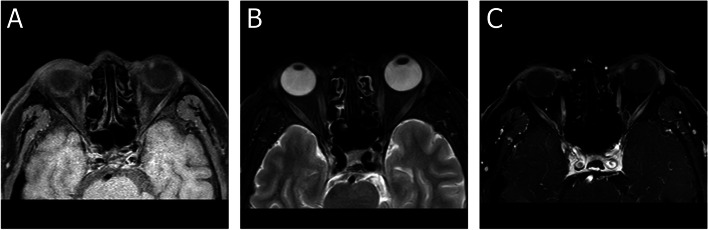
MRI of the eyeball and orbit. In the lens of the left eye, the signal intensity of the mass was slightly hyperintense on T1-weighted images (A) and markedly hypointense on T2-weighted images (B) and enhanced very well (C)
